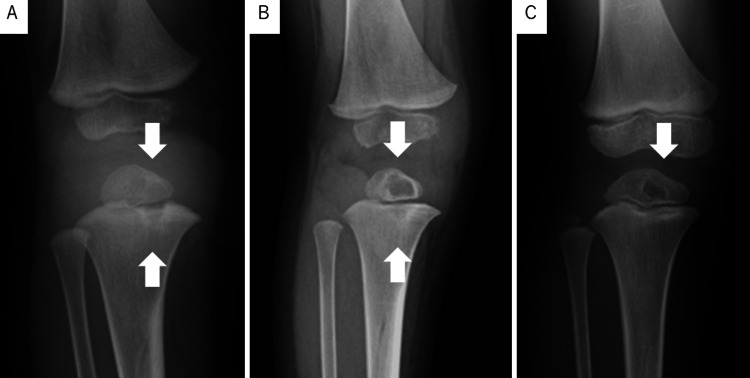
Bone radiography performed from just before the third curettage to the last evaluation A: Just before the third curettage, the lesion expanded (arrow). B: One month after the third curettage, the cavity caused by the curettage could be observed (arrow). C: At the last evaluation, there was no evidence of premature fusion of the epiphysis, and the cavity caused by the curettage shrunk (arrow).
